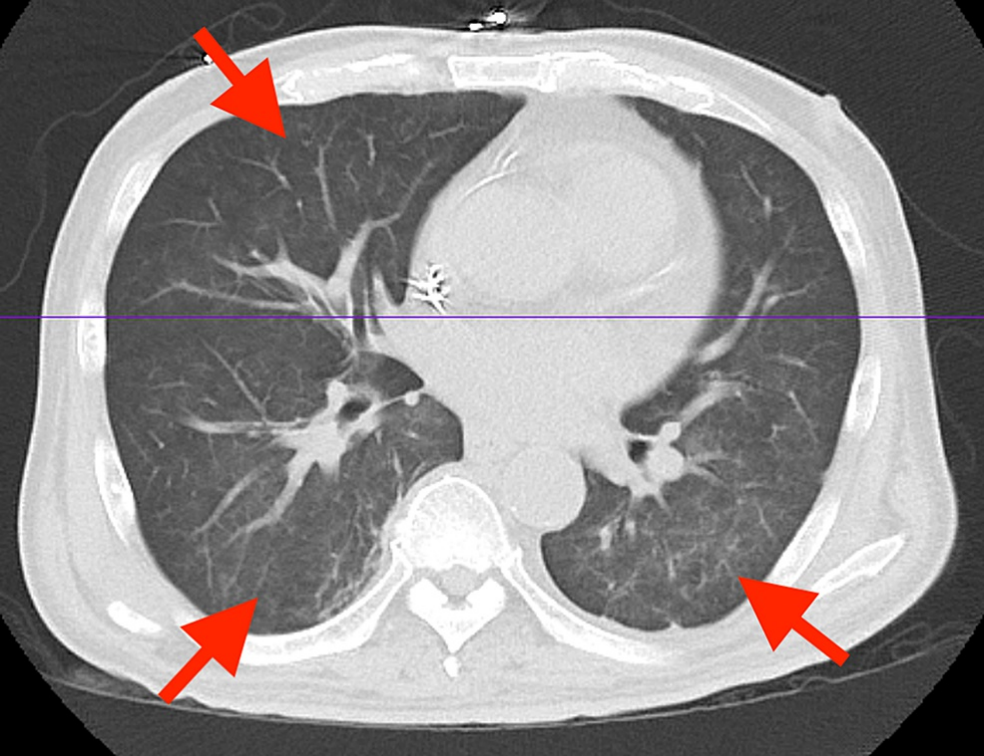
Abdominal and pelvic CT showing mild diffuse bilateral ground-glass opacities, which may reflect infection, inflammation versus pulmonary edema (arrows) CT: computed tomography
